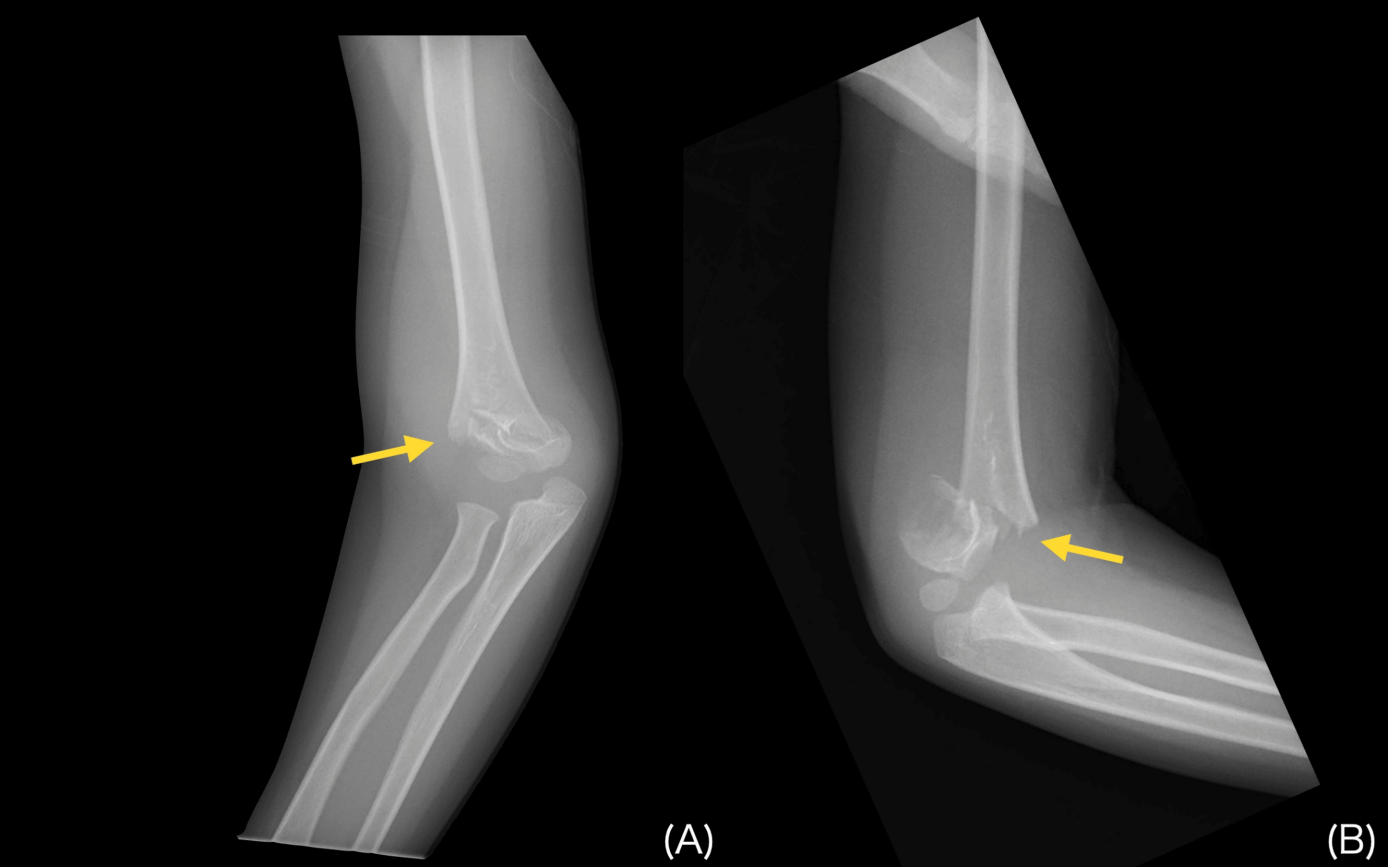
Initial injury radiographs of a displaced supracondylar humerus fracture Initial plain radiographs of the right elbow revealing a displaced supracondylar humerus fracture: anteroposterior view (A) and lateral view (B). Yellow arrows indicate the fracture lines.
